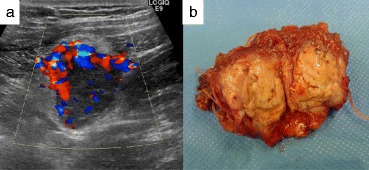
a Ultrasonography image. b Macroscopic findings for the tumor
